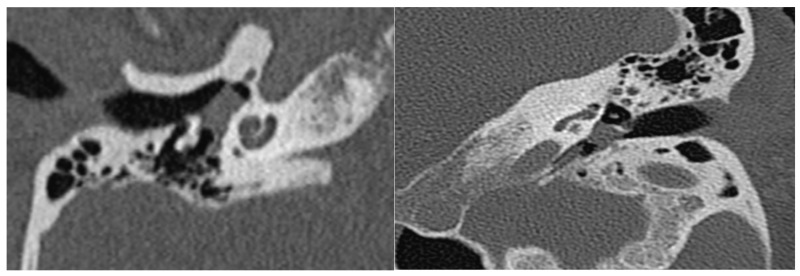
Coronal and axial HRCT sections showing a soft tissue density in the middle ear cavity.
